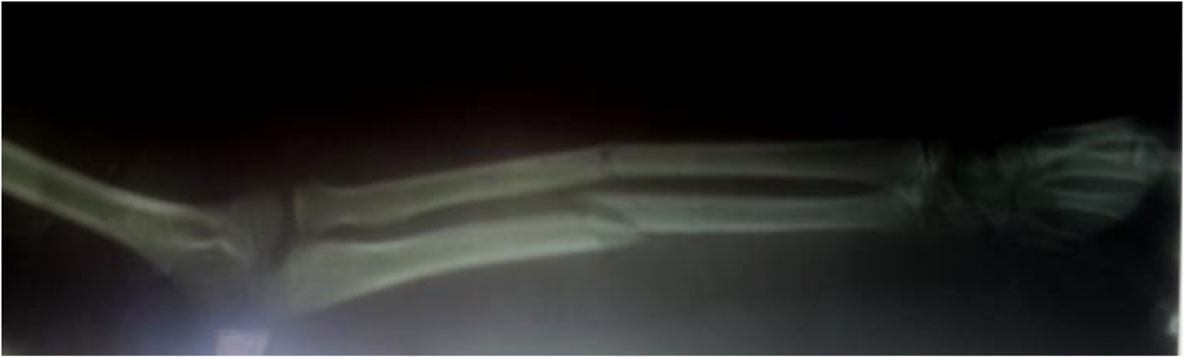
X-ray of left forearm. Fracture of the shaft of the two bones of the forearm classified Orthopaedic Trauma Association/AO 22-A3
